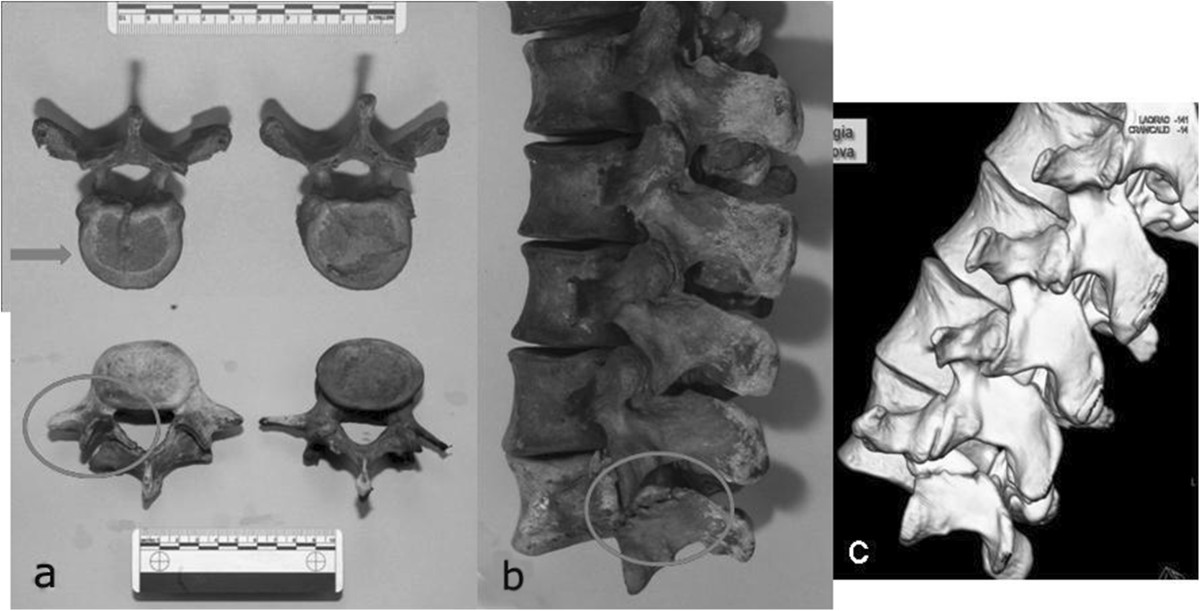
Typical low back disorders were caused in a young horseman by cronic mechanical stresses: a Schmorl’s hearnias and b spondylolysis of the fifth lumbar vertebra, c as well-documented on CT scan.
